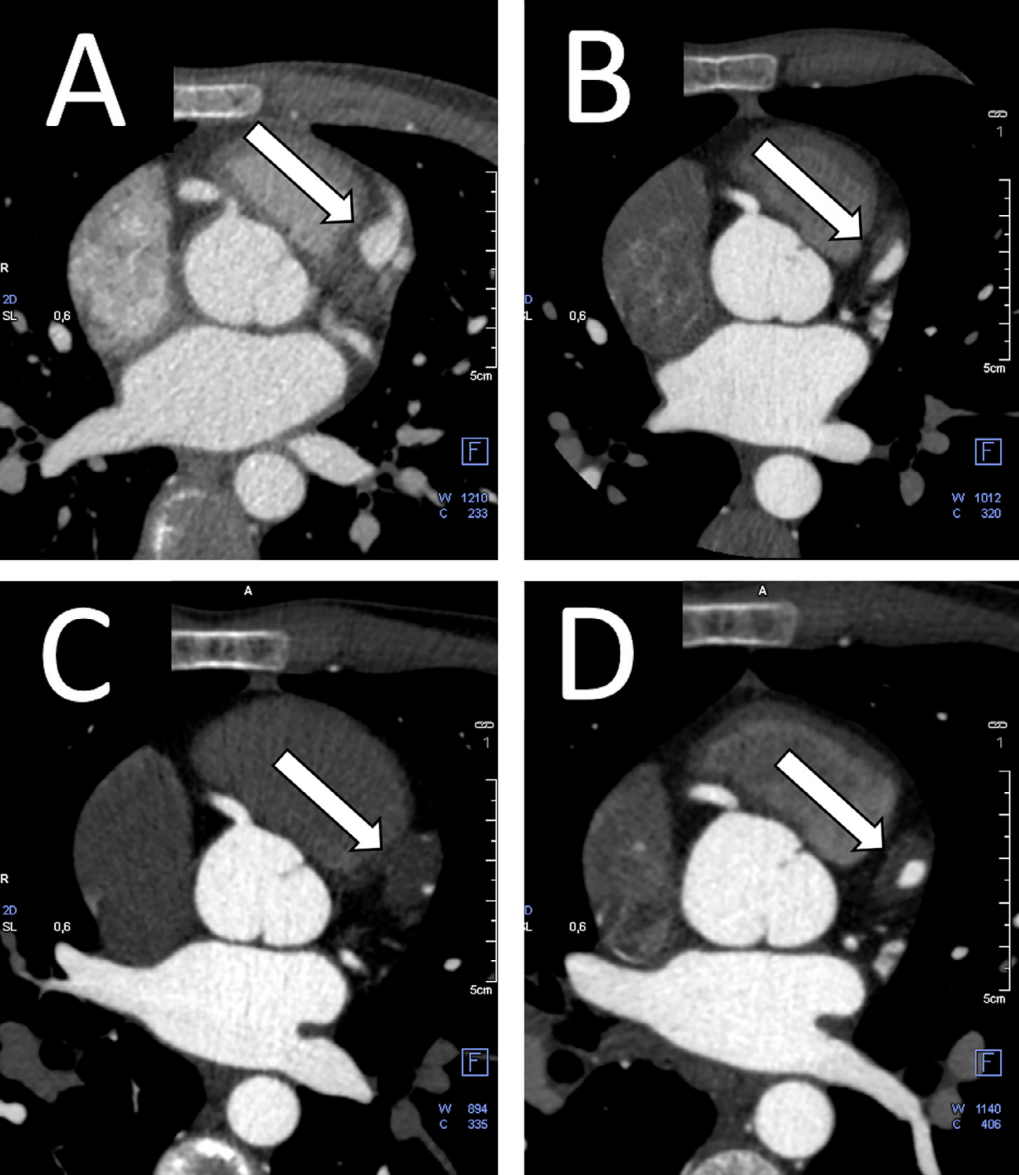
Contrast-enhanced coronary CT showing the progressive thrombosis of the aneurysmal intraventricular artery (A), followed by a thrombosis and decrease of lumen size (B), near occlusion on a follow-up exam 3 years after initial presentation (C), and subsequent improvement (D).
